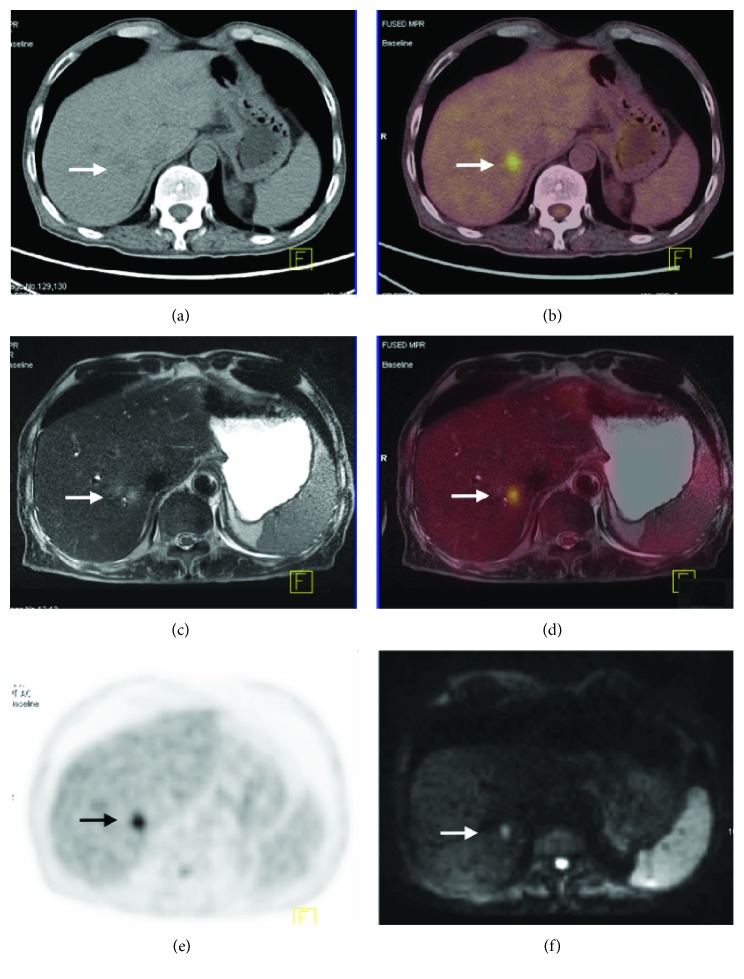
A 75-year-old man with gastric carcinoma diagnosed as liver metastasis proven by clinical information of follow-up. (a) Axial CT image shows suspicious mild low density in segment VII of the liver (arrow). (b, d) On fusion PET/MRI and PET/CT image, the right liver node shows FDG uptake with an SUVmax of 4.3 (arrow). (c) On the T2-weighted axial image, a moderate high signal intensity node is seen (arrow). (e) On the PET image, an obviously avid FDG uptake was observed (arrow). (f) This lesion shows high signal intensity on diffusion-weighted image with a *b*-value of 800 (arrow).
